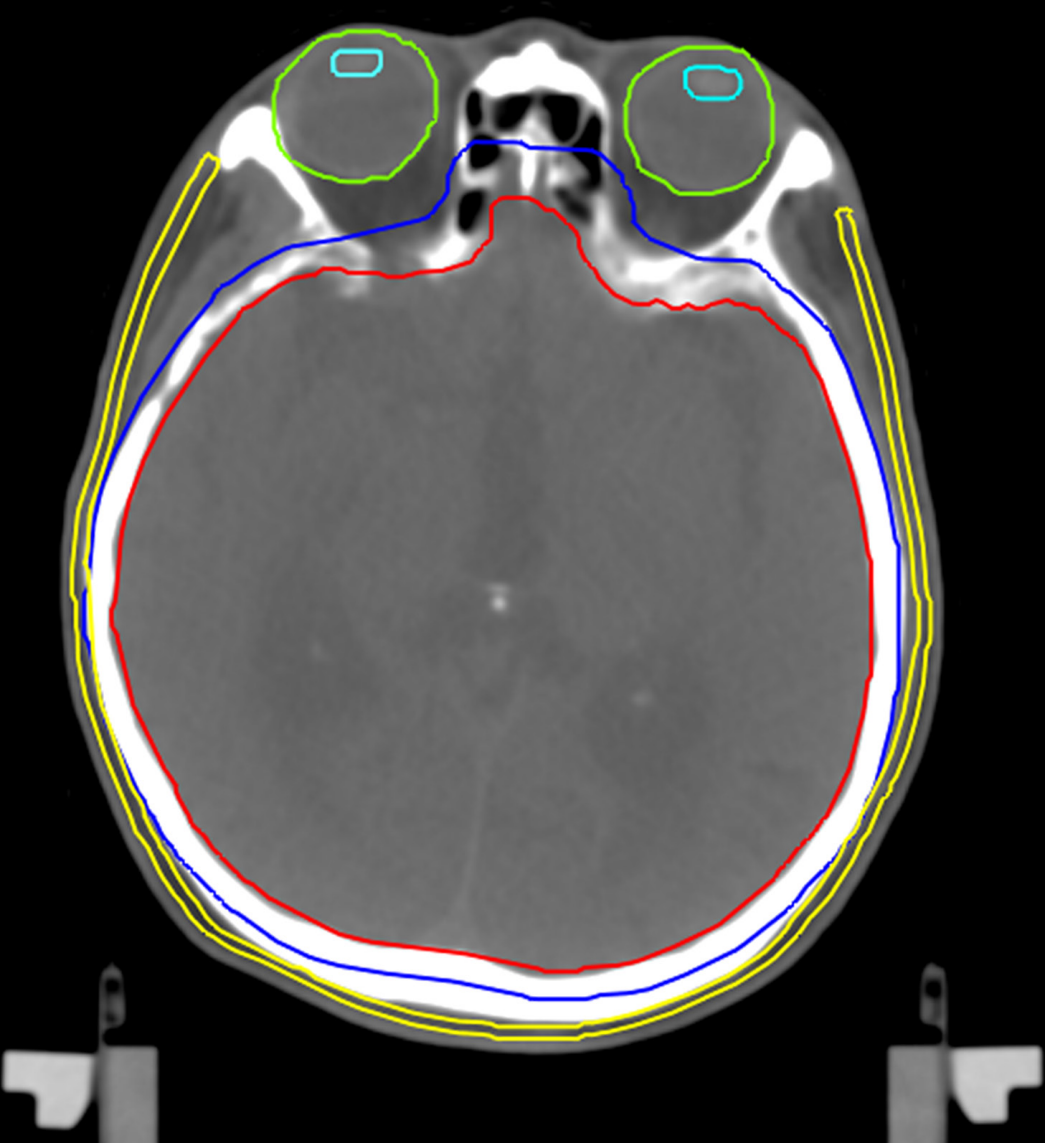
Contoured structures on a CT image. Red and blue lines show the CTV and PTV (5 mm margin), respectively. Yellow, green, and sky-blue lines show the scalp, eyes, and lenses defined as the OARs, respectively. CTV, clinical target volume; OAR, organ at risk; PTV, planning target volume.
